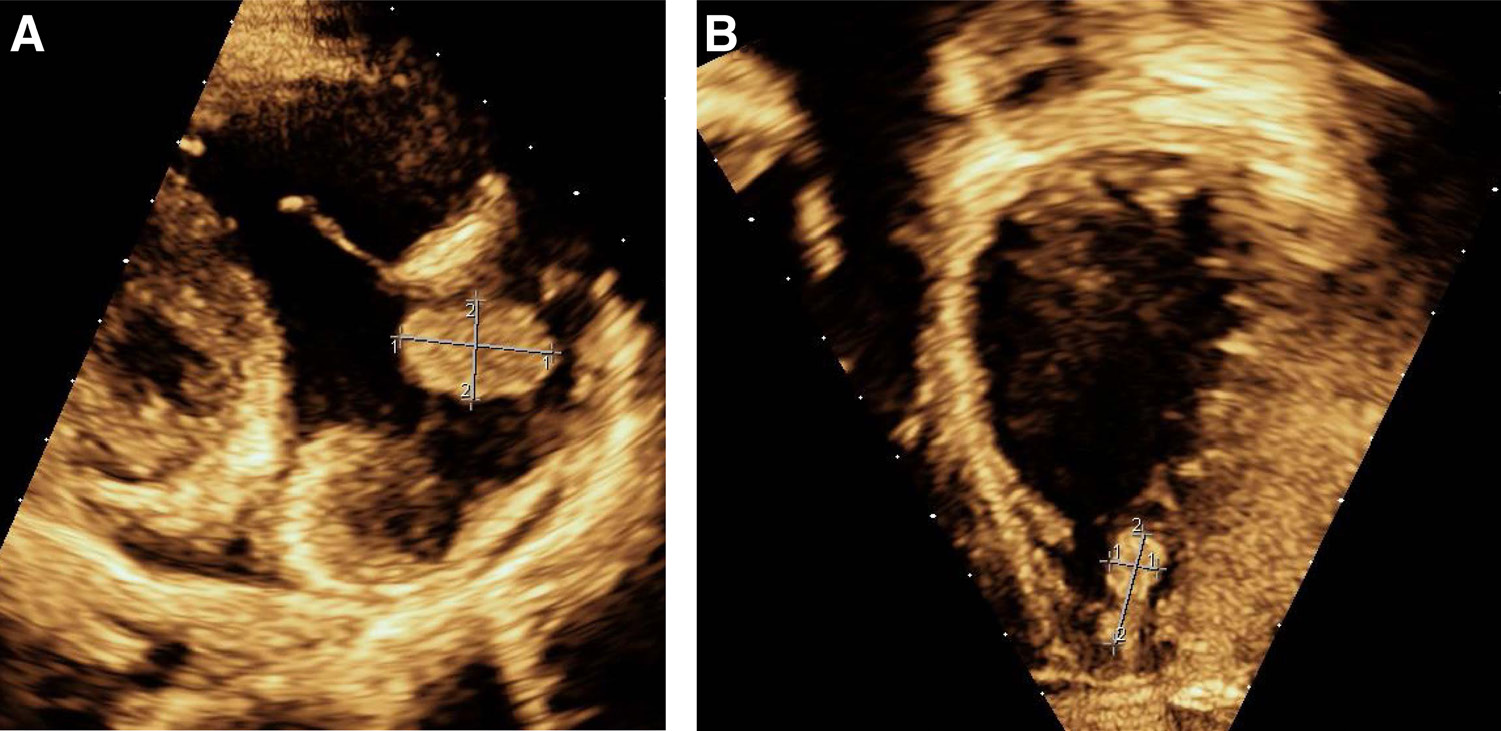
Echocardiogram demonstrating (A) an echogenic mass (2.3 × 1.5 cm) in the right atrium and (B) another echogenic mass (0.7 × 1.6 cm) in the left ventricle.
